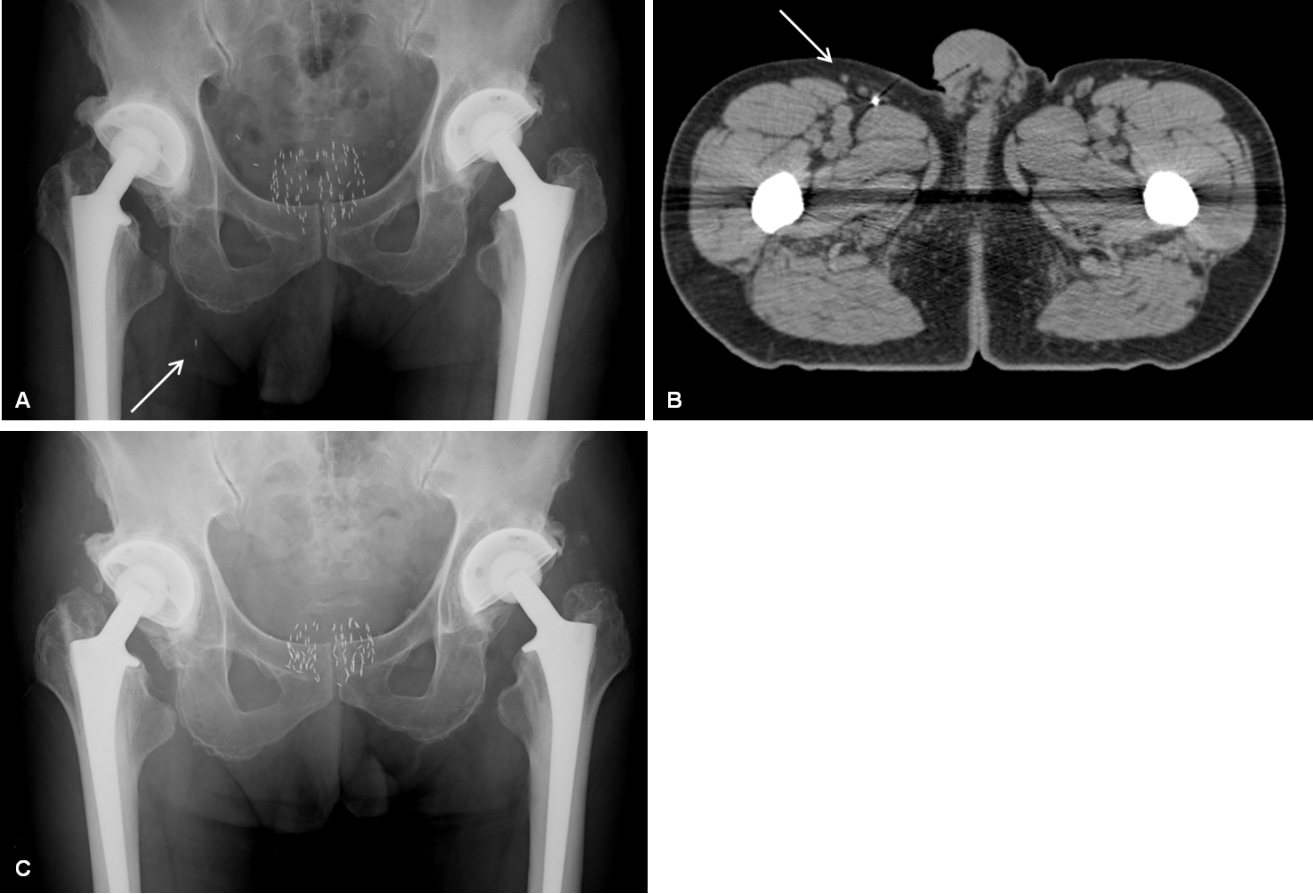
Case 3: Seed disappearance from the right groin area four years after seed implantation. One day after seed implantation, a follow-up pelvic radiograph showed that a seed was located inferior to the right side of the pelvis (solid arrow) (A). Subsequent computed tomography showed that the seed had migrated to the right groin area (solid arrow) (B). Four years after seed implantation, a follow-up pelvic radiograph showed that the seed had disappeared from the pelvic area (C).
